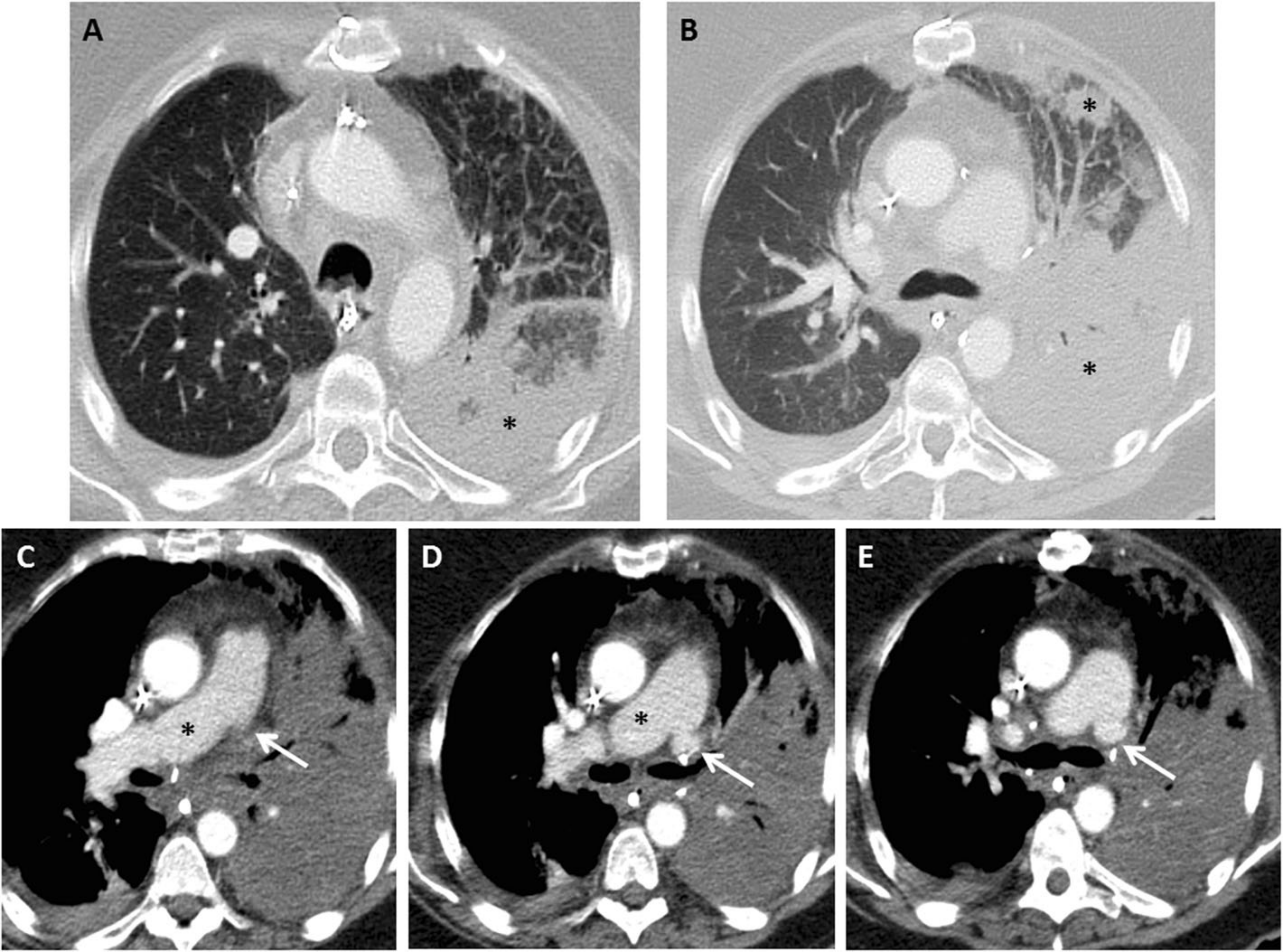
Left pulmonary artery anastomotic occlusion. CT-PE protocol was performed after suspecting left pulmonary artery anastomotic occlusion on initial non-enhanced CT scan performed on day 3 post bilateral lung transplant. Axial images using lung window (a and b) show diffuse and ill-defined consolidations in the left lung, predominantly in the lingula and the left lower lobe (asterisks). These findings are likely related to areas of pulmonary hemorrhage with evolving areas of pulmonary infarcts. There is associated ground glass opacities and interlobular septal thickening, best appreciated in the left upper lobe. Axial images using soft tissue window (c–e) show a total occlusion of the left pulmonary artery at the arterial anastomotic site (arrows) and a patent right pulmonary artery (asterisks). Note the enhancing left pulmonary veins within the left lung consolidation
